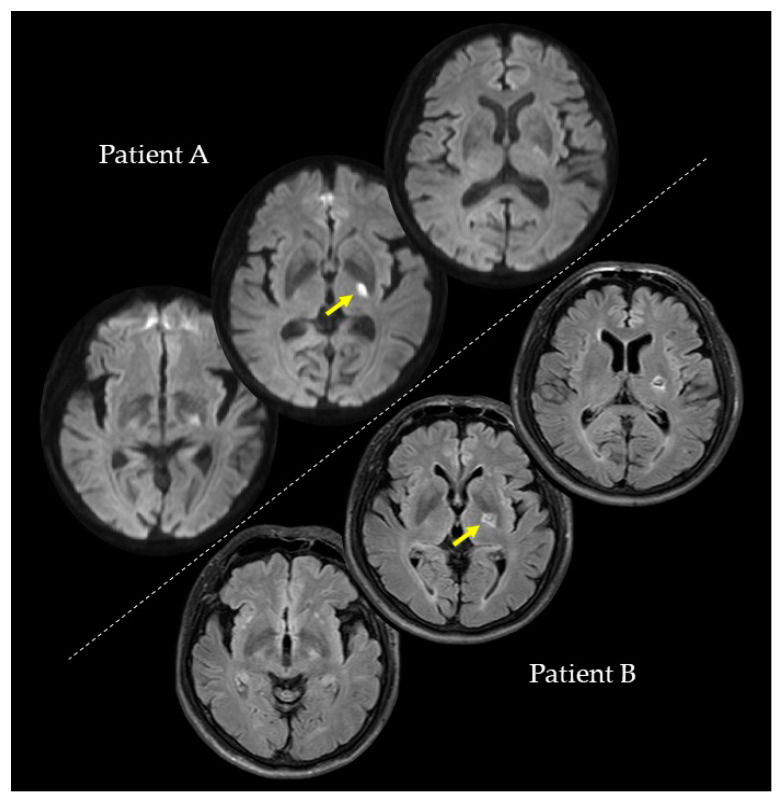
Magnetic resonance imaging scans showing the lesion sites of Patients A and B. Yellow arrows indicate the lesion sites.
